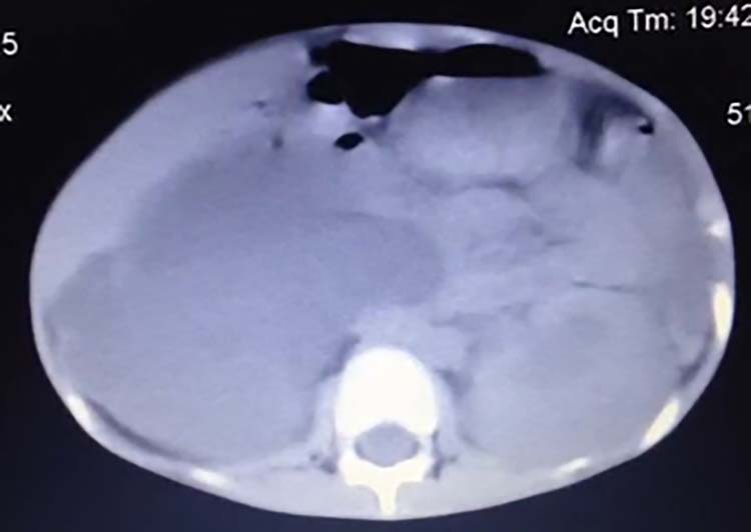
A CT scan of the abdomen and pelvis revealed giant hydronephrosis of the right kidney and mild hydronephrosis of the left kidney.
